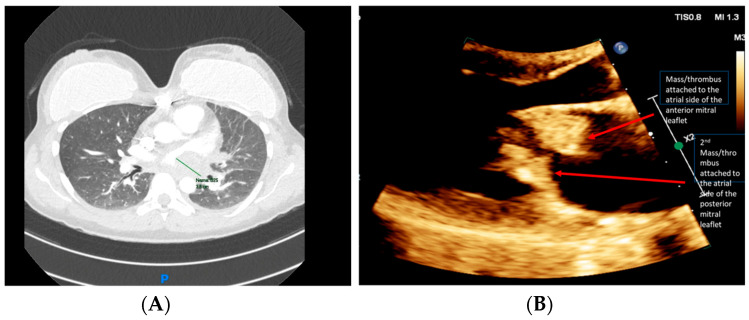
(A) Chest CT revealed a large filling defect extending from the left atrium to the left hilum; and (B) TTE (parasternal long axis) demonstrated multiple mobile masses attached to the mitral valve. Published with the permission of the rights owner.
